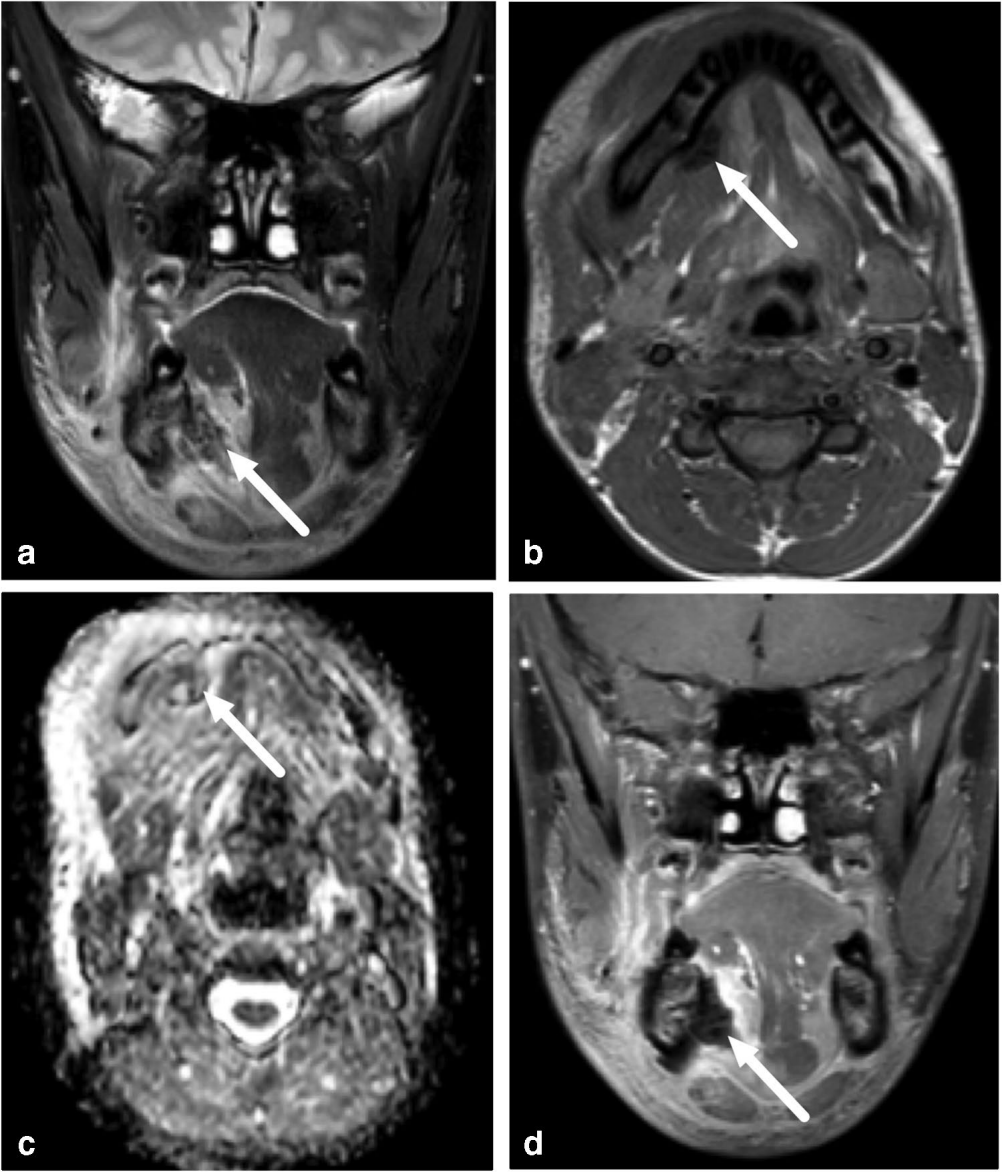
False-negative abscess in a 13-year-old boy with an odontogenic infection. Imaging was done after removal of the infected d46 tooth and incision of an abscess. Coronal fat-saturated T2-weighted (a) and axial T1-weighted (b) magnetic resonance imaging (MRI) images demonstrate extensive soft-tissue edema, and a relatively T2 and T1 hypointense subperiosteal collection (*arrows*). Axial apparent diffusion coefficient images (c) and post-contrast coronal fat-saturated T1-weighted images (d) show no restricted diffusion or contrast enhancement (*arrows*). This heterogeneous subperiosteal collection was considered to contain postoperative gas and/or blood with no clear evidence of a residual abscess. Yet, purulence was found at surgery
